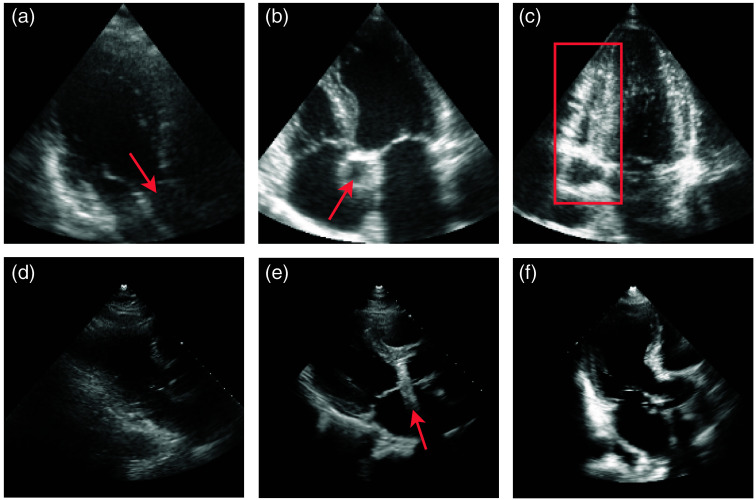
Explainable misclassification examples from CAMUS^27^ (a)–(c) and EchoNet-LVH^28^ (d)–(e). (a) Apical three-chamber view with two-chamber reference label (arrow: outflow tract). (b) Apical five-chamber view with four-chamber reference label (arrow: ascending aorta). (c) Poorly optimized apical four-chamber view (right, bounding box: right heart). (d) and (e) PLAX view inferred as unknown by proposed method (arrow: imaging artifact). (f) Apical three-chamber view with PLAX reference label.
